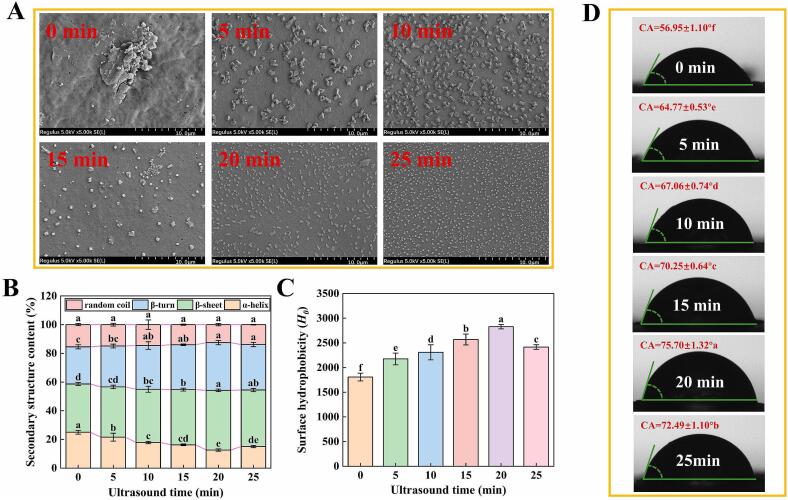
Microstructure (A), secondary structure (B), surface hydrophobicity (C) and three-phase contact angle (D) of water-dispersible PSE-like myofibrillar protein microgel particles (MMPs) prepared with different ultrasound times (0, 5, 10, 15, 20, and 25 min). Different letters (a–f) above error bars indicate significant difference (*p* < 0.05) among different samples.
